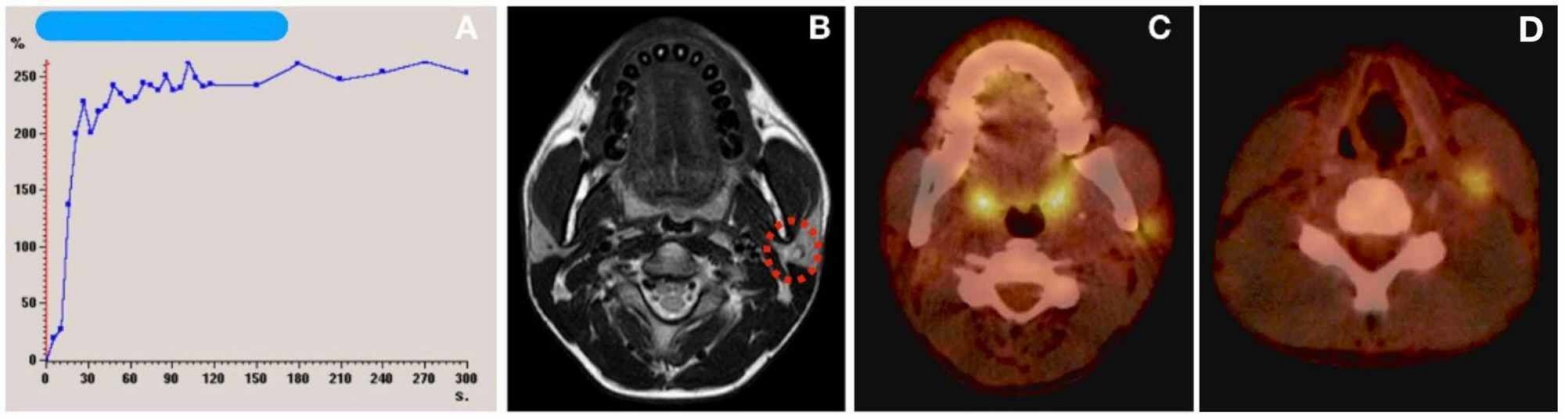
A) DWI demonstrate a type III ADC curve with an early rise and moderate contrast wash suggesting of malignancy; B) T2 weighted image MRI with a lesion in the left parotid mass - red dotted circle (1.5-T system; Ingenia, Philips Healthcare Systems, The Netherlands); C) PET-CT scan with a left parotid mass; D) PET-CT scan with a lymph node in the level II DWI: diffusion weight image; ADC: apparent diffusion coefficient; PET-CT: positron emission tomography CT
